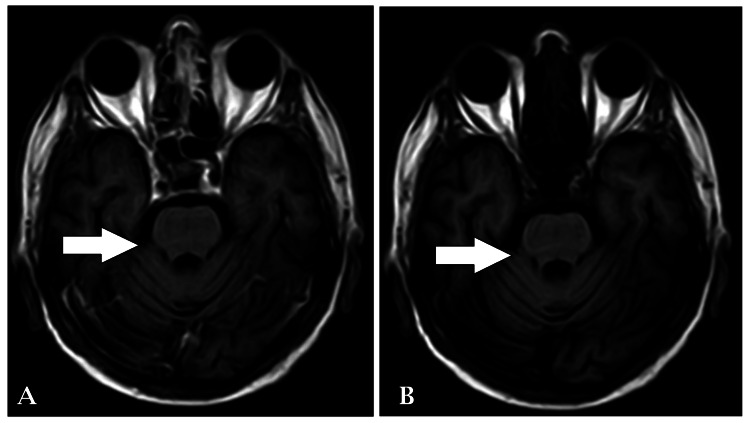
T1WI and T1+C axial section of brain showing non-enhancing lesion in pons T1WI: T1-weighted imaging; T1+C: T1-weighted imaging with contrast
